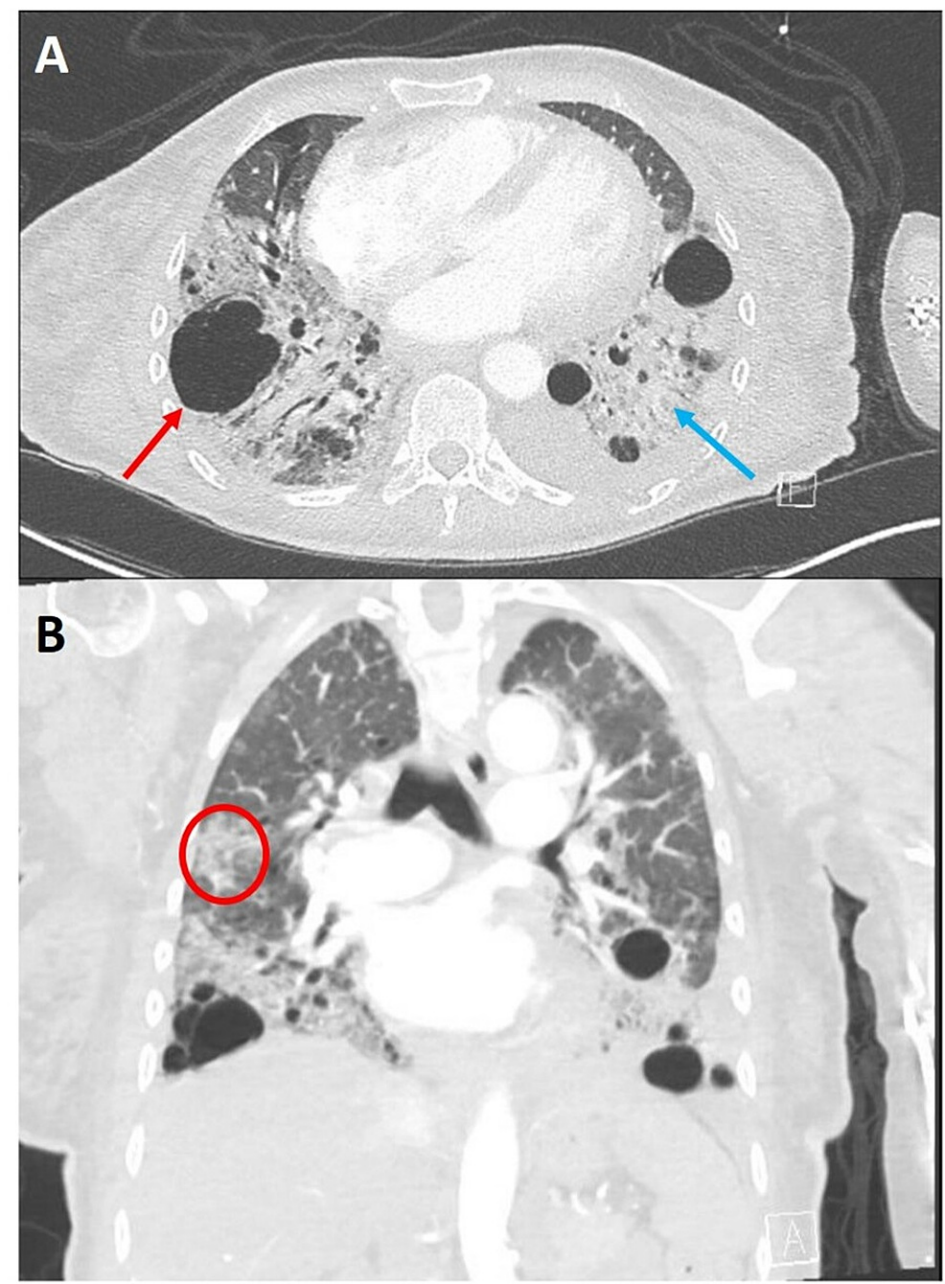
Representative axial (A) and coronal (B) CT angiography images of patient’s current admission. Axial images demonstrate interval progression of heterogenous cysts (red arrow), some of which appear to have coalesced into bullae. Cysts appear to be distributed peripherally. Coronal cuts demonstrate a craniocaudal gradient. Also seen are ground-glass opacities (red circle), dense consolidations (blue arrow), a left-sided pleural effusion, and air bronchograms.
